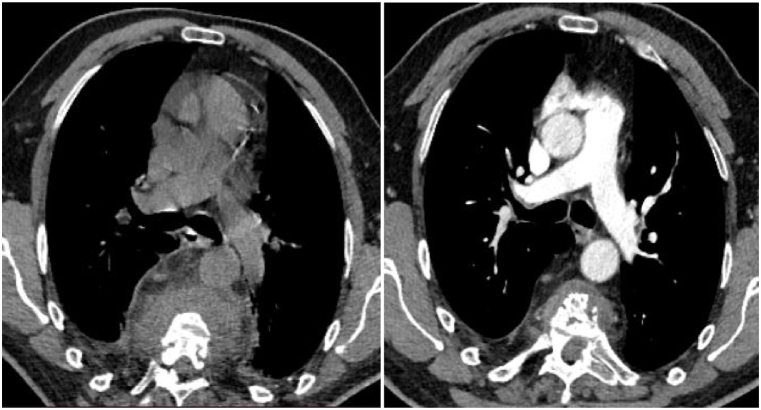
CT scan cross-section at T6 vertebra (2020 on the left and 2022 on the right).
